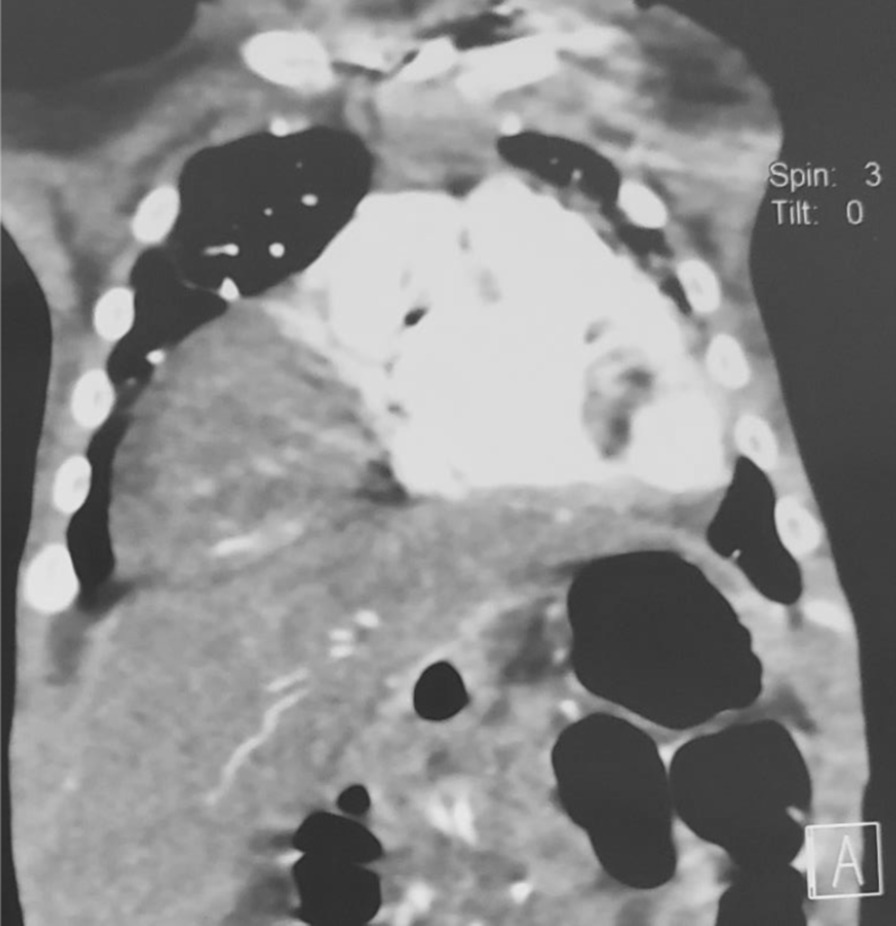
Front view of the thorax on contrast-enhanced computed tomography demonstrates focal elevation at the medial portion of right hemidiaphragm containing abdominal viscera
